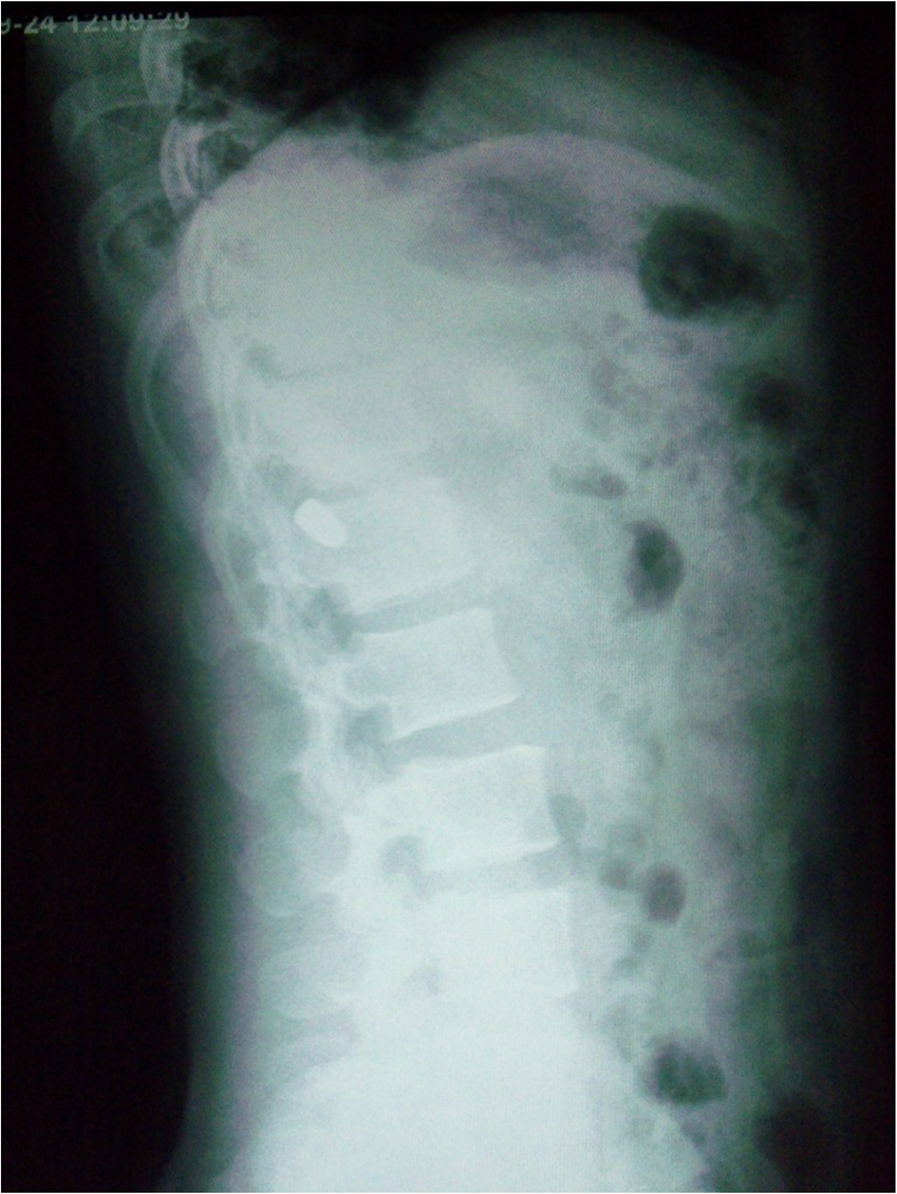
Lateral abdominal radiograph of the patient. Radiograph showing bullet to be lateral to the spine.
